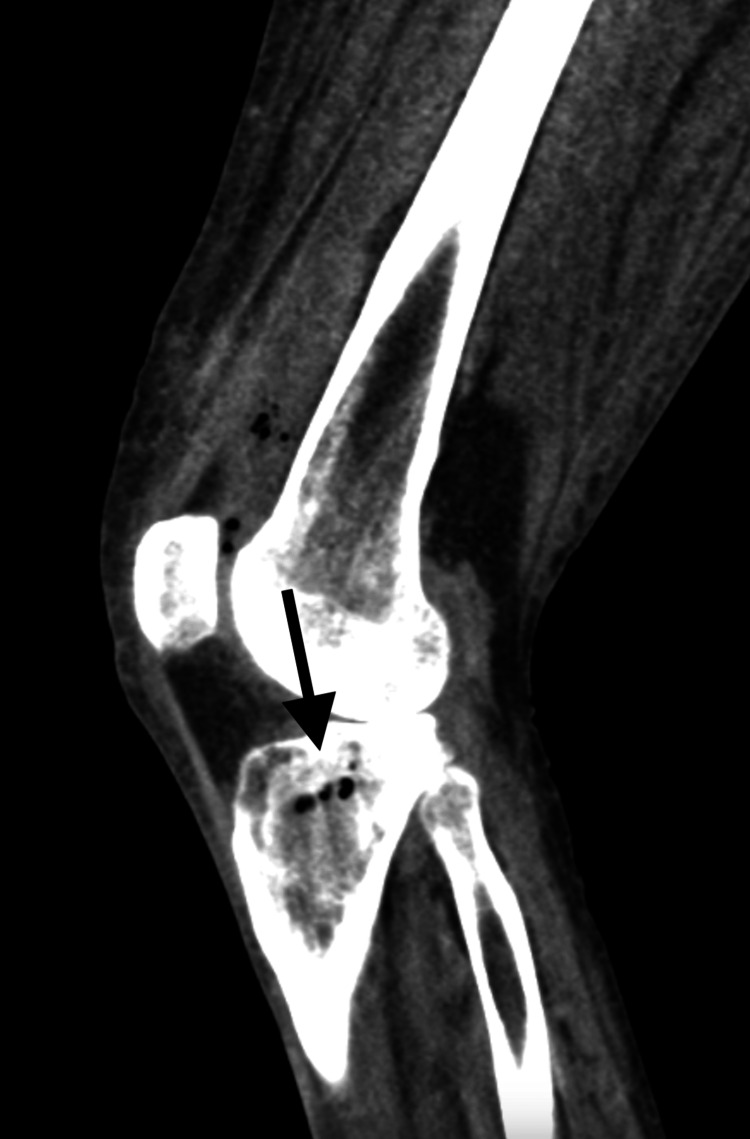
Emphysematous osteomyelitis of the left proximal tibia. Sagittal CT of the left knee joint in a 45-year-old man with knee pain reveals emphysematous osteomyelitis of the left proximal tibia (black arrow).
